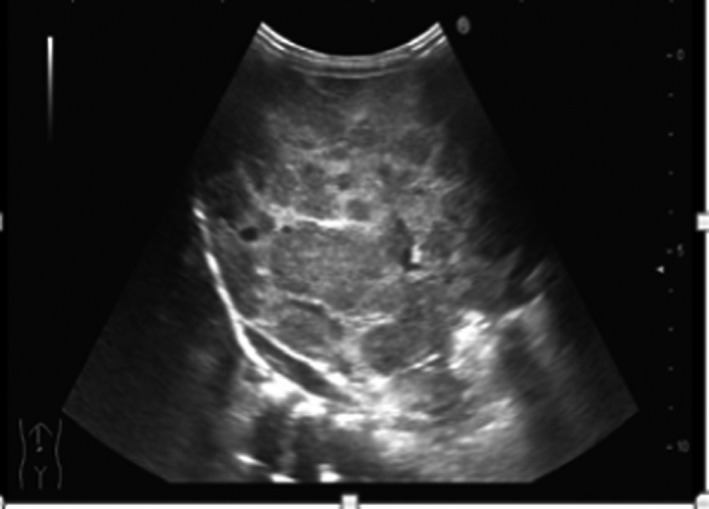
Abdominal ultrasound showing huge diffusely heterogeneous hepatomegaly 13 × 8 ×14.6 cm (volume 974 mL) with well‐delineated innumerable lesions (5–42 mm of diameter) hypoechogenic, some with peripheral halo displacing the adjacent vessels.
